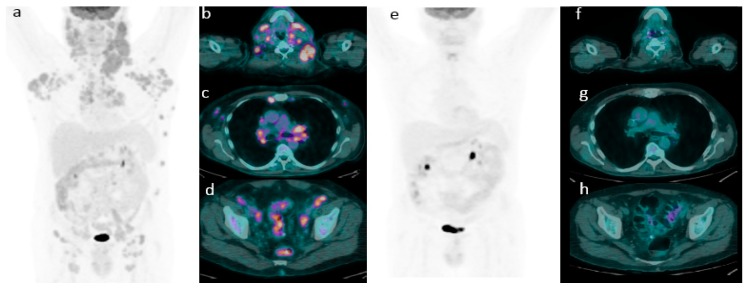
Emblematic example of complete metabolic response. A representative case of a 56-year-old male with stage III MCL. (a) Baseline maximum intensity projection (MIP), showing diffuse hypermetabolic disease in (b) laterocervical, (c) mediastinal and (d) iliac nodes. (e) PET/CT after chemotherapy showing a complete metabolic response (Deauville score 1) with no 18F-FDG uptake (f–h) with the disappearance of previous lesions.
